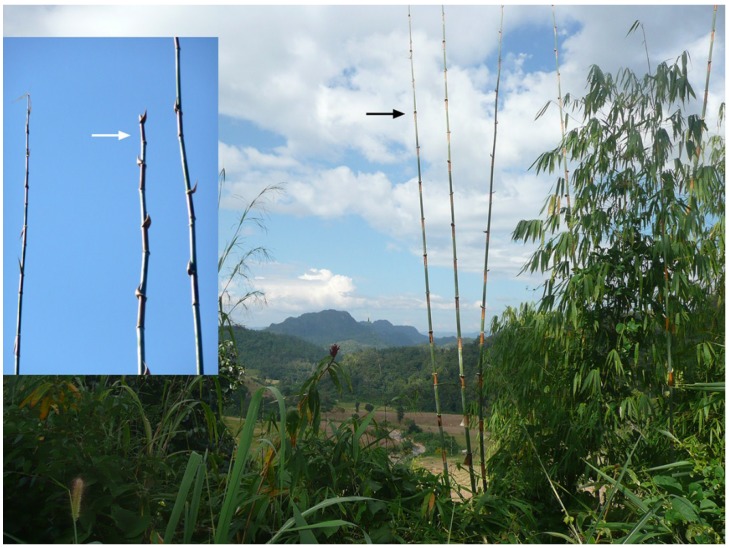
Habitat of *A. rufipes* in November (N Thailand, Pangmapha district). The black arrow indicates a bamboo shoot of *Cephalostachyum pergracile*, the host plant of *A. rufipes*; Inset: *A. rufipes* larvae inhabit the fifth to sixth internode below the apex of a bamboo shoot. After some time, the apical four to five internodes die off and fall to the ground, whereas the infested internode (white arrow) remains on the top of the shoot for a longer time.
